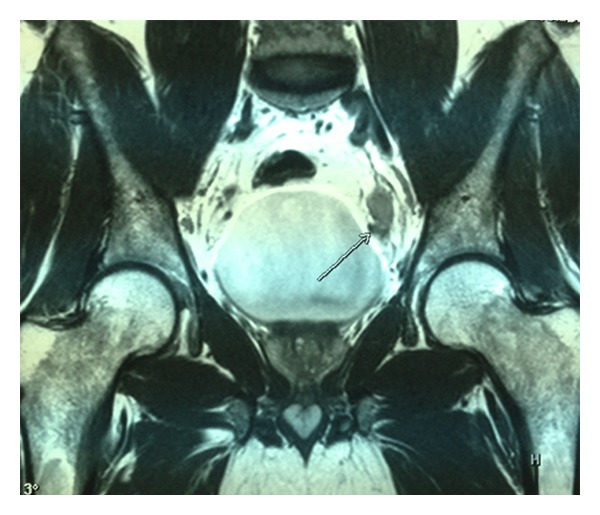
Coronal MR image demonstrating a solid mass located in the left distal ureter.
